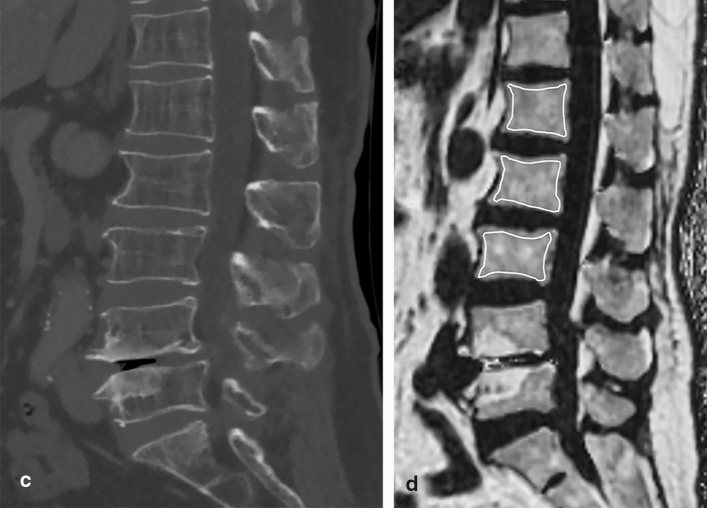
DXA scan, CT scan, and MR PDFF map of a 69-year-old female patient. (a) The T-score of L1-4 in the DXA scan is − 3.2, − 3.0, − 2.9, and − 0.5, respectively. Since the T-score of L4 increased by more than 1 SD than that of L3, it is excluded from the measurement. Therefore, the BMD of this patient is measured to be 0.774 g/cm^2^, which is the mean of L1–3. (b) TBS is measured as 1.369 as the average value of L1–3 selected for BMD measurement. (c) A sagittal CT image show sclerotic changes around the lower endplate of L4. HU measurements are performed in L1–3, and the mean HU values are 54.71 (d) PDFF of L1–3 bodies are measured in the MR PDFF map, showing the region of interest to measure PDFF in each body. PDFF are 62.98. DXA—dual-energy X-ray absorptiometry, PDFF—proton density fat fraction, SD—standard deviation, BMD—bone mineral density, TBS—trabecular bone score, HU—Hounsfield unit.
